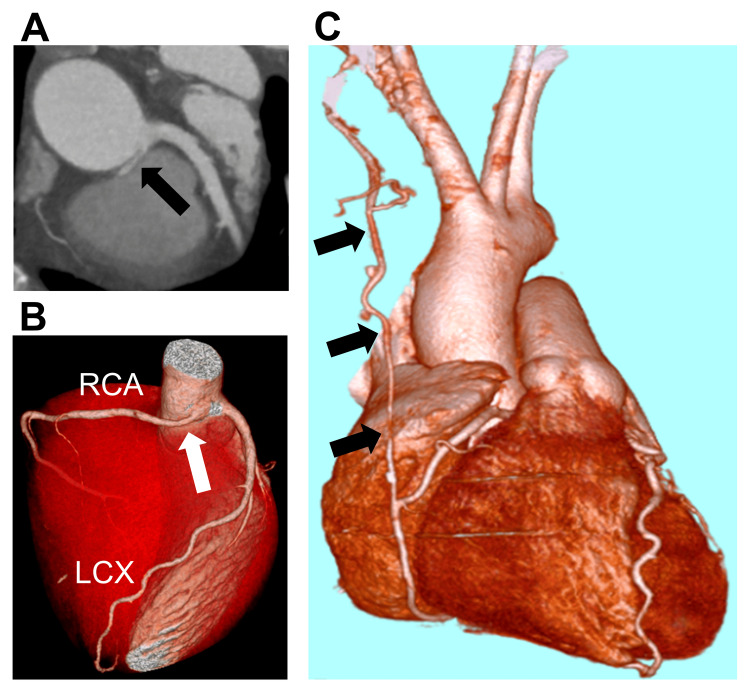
(A) Two- and (B) three-dimensionally enhanced computed tomography scan showing an anomalous origin of the RCA from the left sinus of Valsalva with an intramural course. (C) Bypass of the blocked RCA using the RITA. (A) Black and (B) white arrows indicate the origin of the RCA. (C) Black arrows indicate the bypass graft of the RITA to the RCA. RCA = right coronary artery; RITA = right internal thoracic artery; LCx = left circumflex artery
